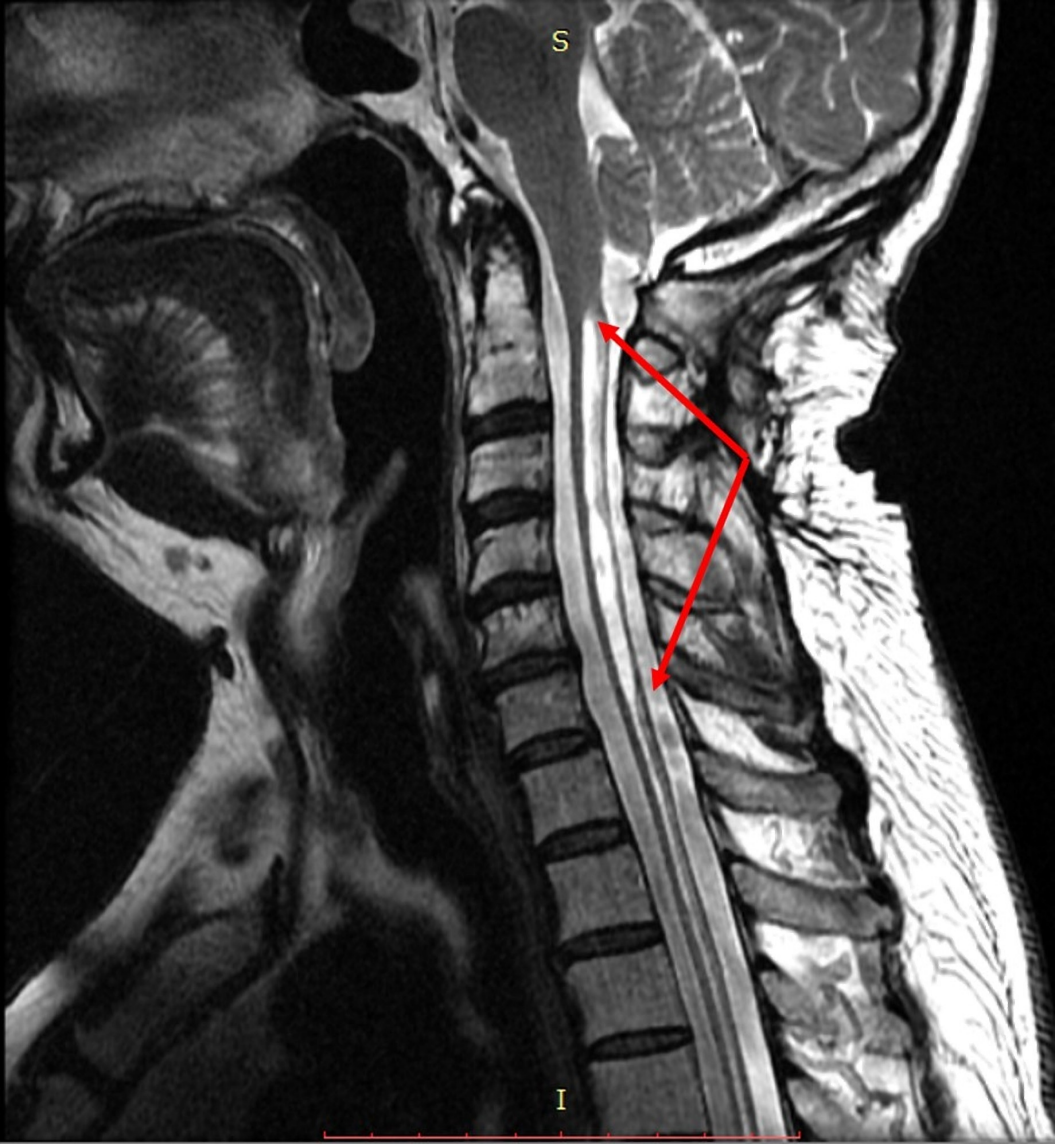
Sagittal T2-weighted magnetic resonance imaging (MRI) of the spine Showing syrinx formation (hyperintense region) from C2-T1, with the greatest dilation around C2-C7 (red arrows).
